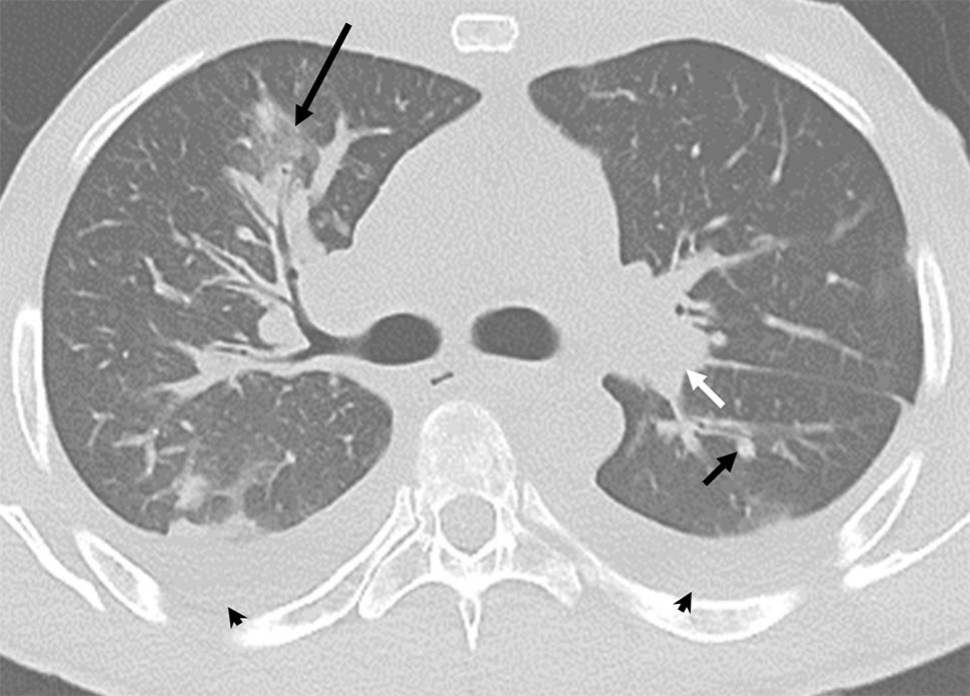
29-year-old man with KS and HIV. Axial CT showing nodular opacities (short arrow) and ground-glass halos (long arrow) surrounding the bronchovascular bundles. Lymphadenopathy (short white arrow) and bilateral pleural effusions (arrowheads) are also noted
